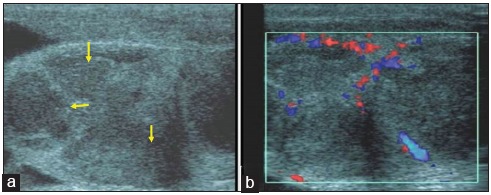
A 44-year-old man with testicular tuberculosis who presented with 6 months history of testicular pain. (a) Gray scale sonographic image demonstrates an enlarged and heterogeneous testis with the presence of multiple ill-defined focal hypoechoic lesions (small yellow arrows). (b) Color Doppler shows increased vascularity along the periphery of the hypoechoic lesions. These imaging findings are non-specific and may be seen in both inflammatory and neoplastic conditions. A diagnosis of testicular tuberculosis was made the following orchiectomy.
